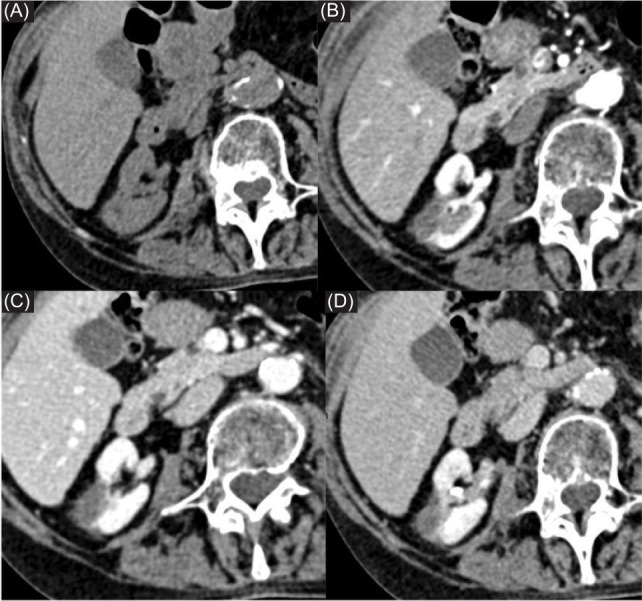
Contrast-enhanced CT scan after 6 months demonstrated complete necrosis area without residual viable tissue.
